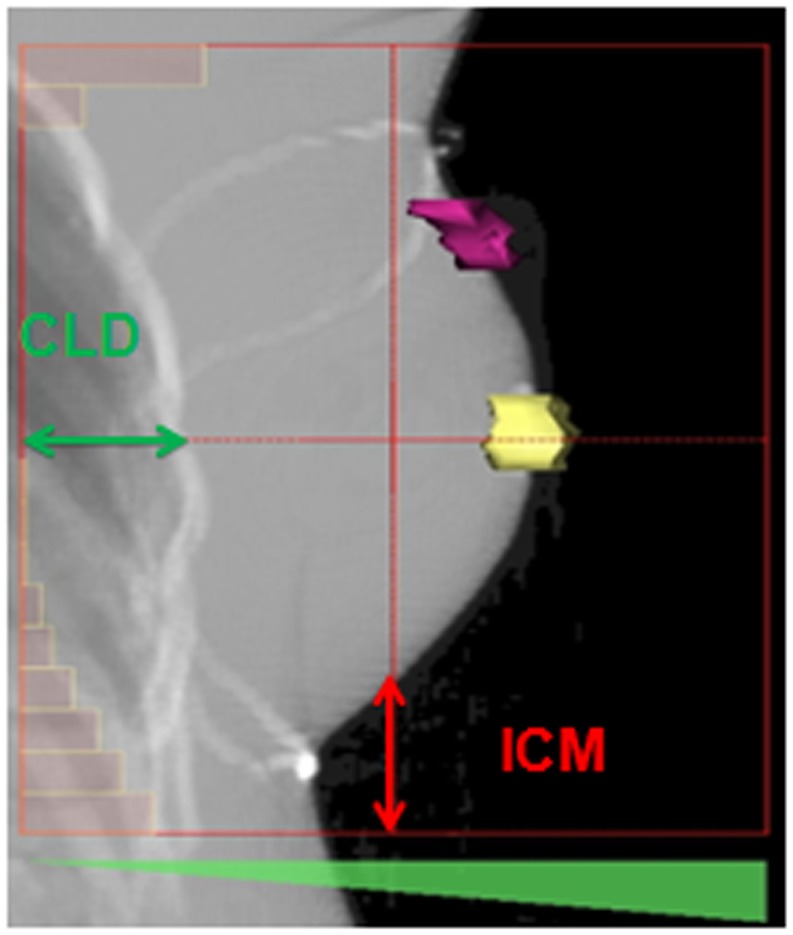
Description of the inferior central margin and central lung distance on a digital reconstructed radiography performed on the treatment planning system Isogray^®^ according to Fein et al. (6) and Kong et al. (10). The nipple and the surgical scar are, respectively, delineated in yellow and purple. CLD, central lung distance; ICM, inferior central margin.
